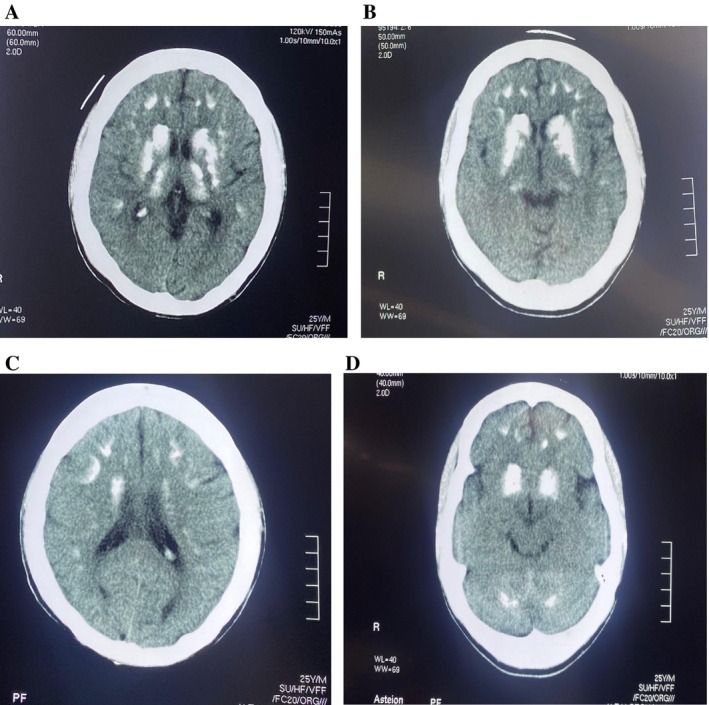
(A) CT brain revealed bilateral calcification in Basal ganglia, thalami, and cerebellar dentate nuclei. (B) CT brain revealed bilateral calcification in Basal ganglia and the Thalamus. (C) CT brain revealed bilateral calcification in Subcortical white matter, basal ganglia and periventricular region. (D) CT brain revealed bilateral calcification in Basal ganglia particularly the globus pallidus and cerebellar dentate nuclei.
